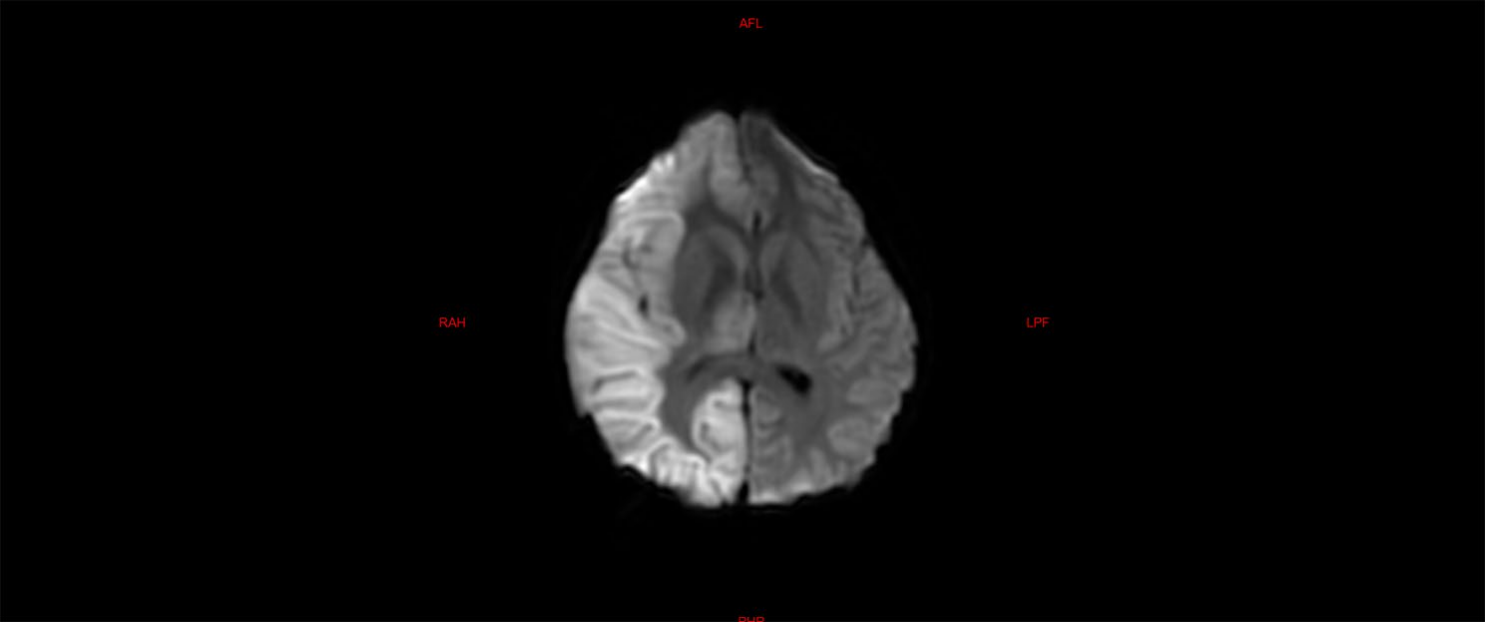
Brain MR DWI sequence of case 7, showing diffuse swelling of the right cerebral hemisphere, shallow sulcus fissure, diffuse diffuse high signal, patchy diffuse high signal in the right basal ganglia and thalamus, and pressure change of the right lateral ventricle Flattened, the midline structure is slightly shifted to the left. Upon admission, the patient developed edema in the right hemisphere of the brain, along with damage to the right basal ganglia and thalamus. Despite treatment, necrotizing encephalopathy was still considered. Although the patient was successfully weaned off advanced life support, severe motor and cognitive impairments persisted
